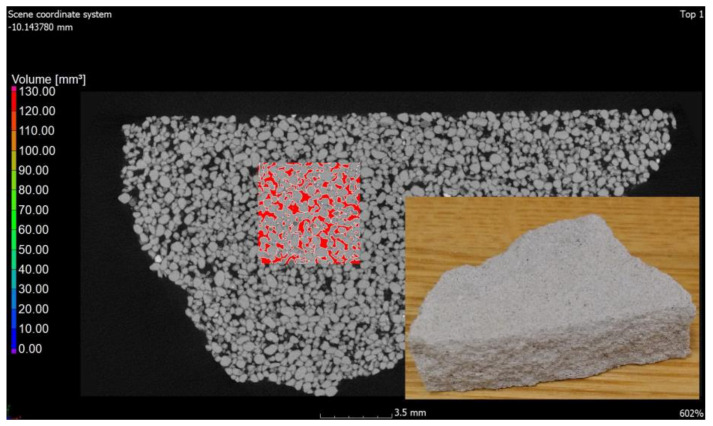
Photo of traditional material (reference sample) examined with computed tomography.
